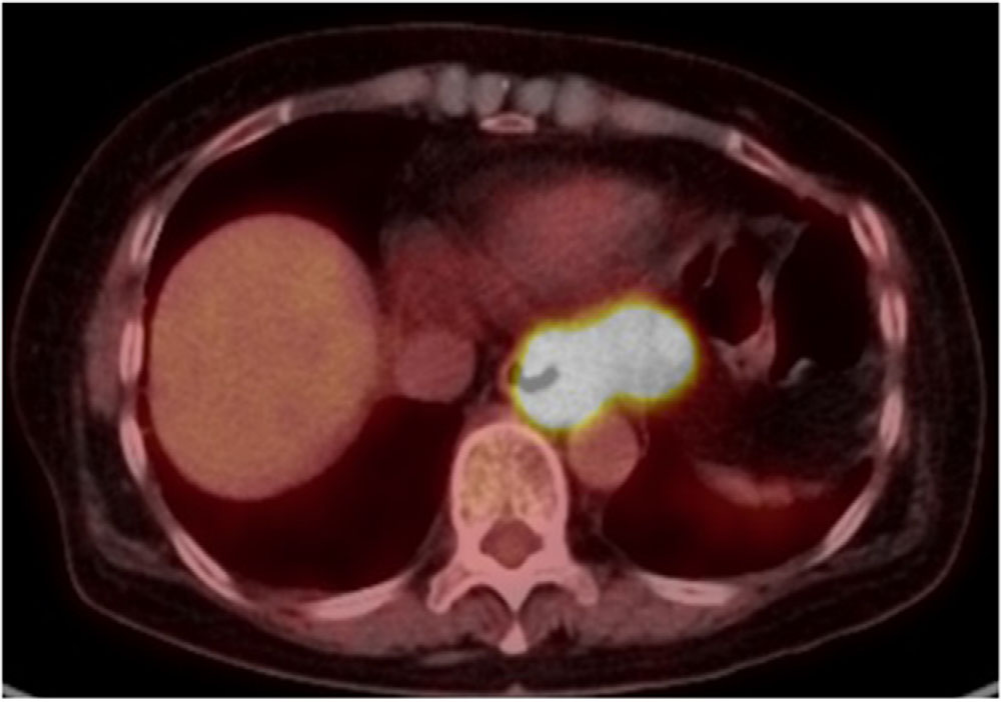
Positive emission tomography–computed tomography scan shows focal uptake from the lower esophagus to the gastroesophageal junction.
